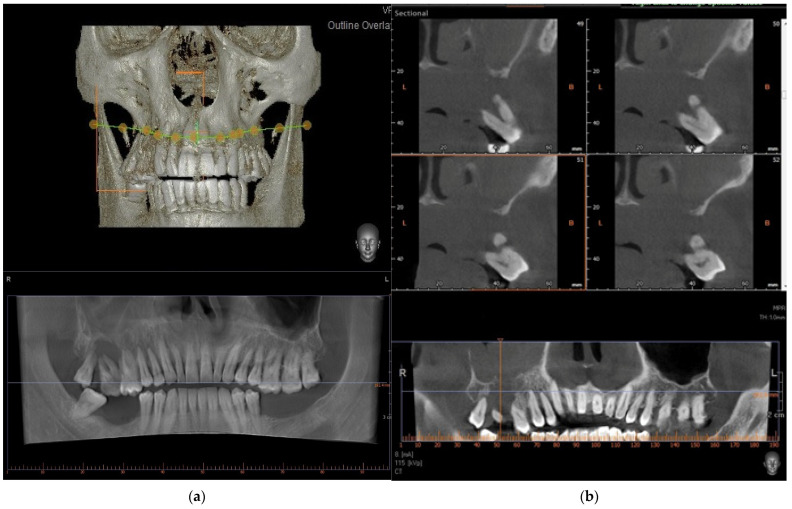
Preoperative radiograph images. In image (a) no bone destruction is detectable in the 3D reconstruction. Below, a simil panoramic image shows bone destruction in the upper right molar region (a). CBCT reveals the osteolytic change in the upper molar region, involving the hard palate (right) (b).
